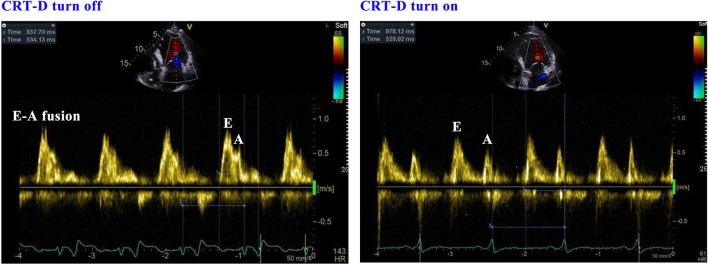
The response of echocardiography when the CRT-D is turned on and off. CRT-D, cardiac resynchronization therapy with defibrillation.
